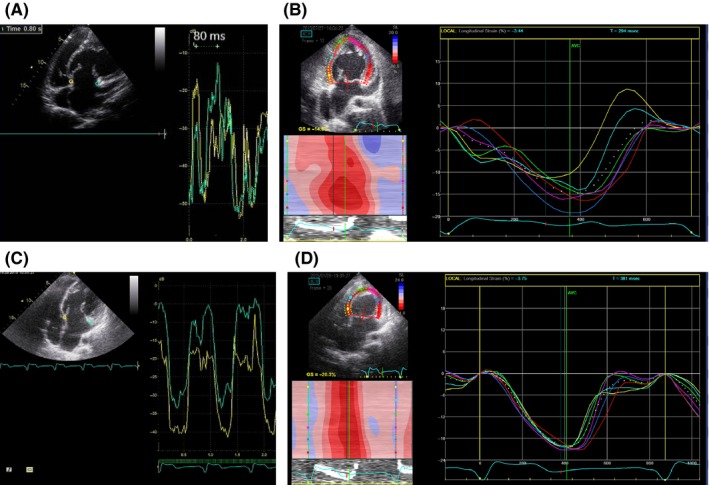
Tissue Doppler imaging and 2‐dimensional (2D) speckle tracking echocardiography before and after cardiac resynchronization therapy (CRT). A, Pre‐CRT tissue Doppler imaging. An intraventricular delay of 80 ms was observed between the end of the right ventricular (RV) free wall contraction and the end of the septal wall contraction on tissue Doppler imaging. B, 2D speckle tracking echocardiography under temporary subpulmonary ventricular pacing. 2D speckle tracking echocardiography showed intraventricular dyssynchrony in the systemic right ventricle. C, Post‐CRT tissue Doppler imaging. After CRT, the intraventricular delay between the end of the RV free wall contraction and the septal wall contraction was improved on tissue Doppler imaging. D, Post‐CRT 2D speckle tracking echocardiography. After CRT, 2D speckle tracking echocardiography showed the disappearance of intraventricular dyssynchrony in the systemic right ventricle
